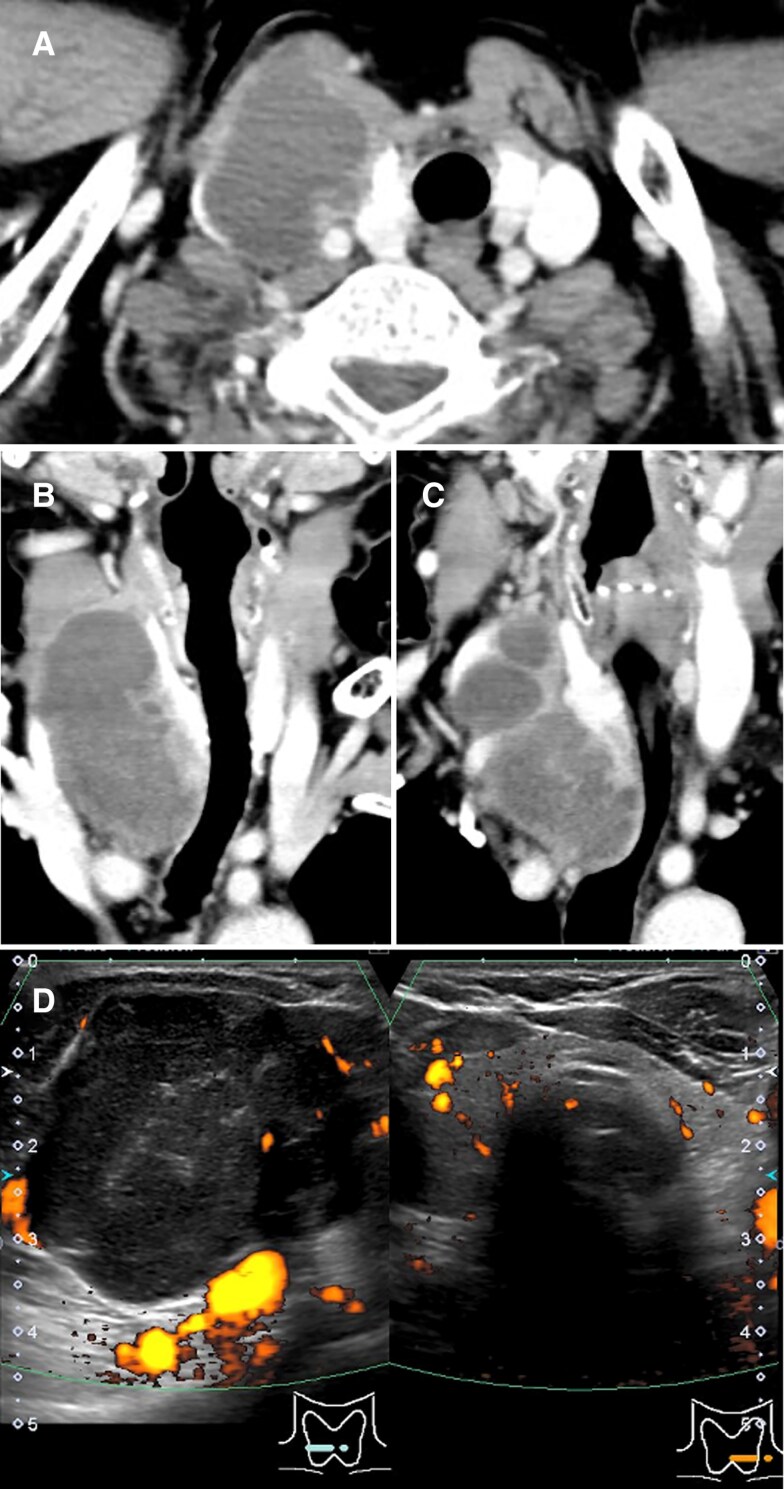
Cervical computed tomography (A, B, C) and color Doppler ultrasonography (D) at presentation. The main locus of the polycystic tumor was the lateral aspect of the thyroid gland.
